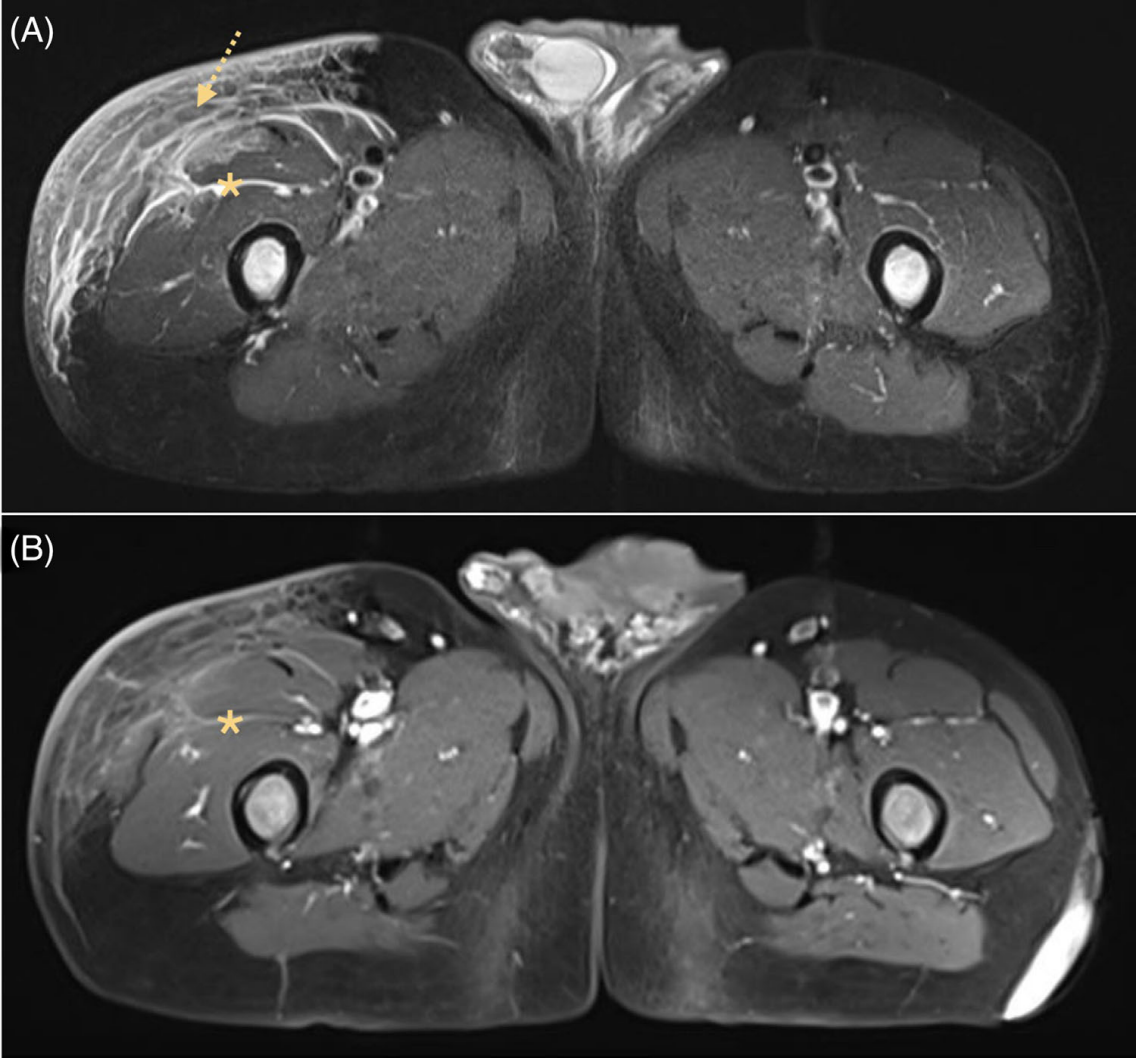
Magnetic resonance imaging (MRI) of upper legs. Signs of fasciitis of the right thigh. Panel (A): short TI inversion recovery (STIR). Increased signal intensity in muscle of the right thigh (*). Marked skin thickening with signal hyperintensity and trabeculation of subcutaneous fat (arrow). Panel (B): fascial enhancement in contrast sequences (*)
